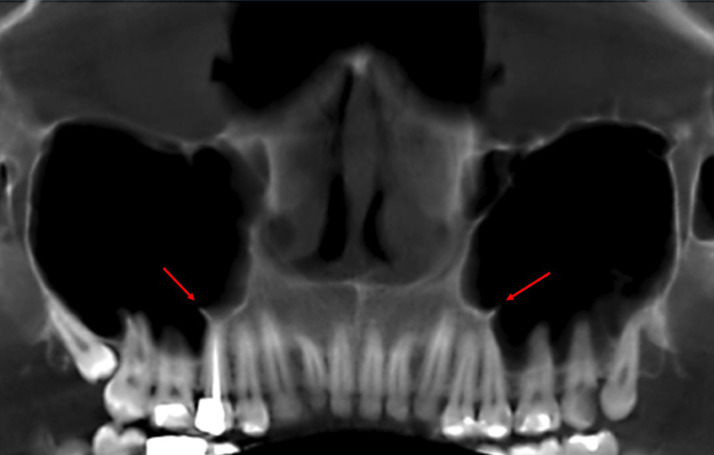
Panoramic-like view of cone-beam computed tomography showing bilateral partial septa.
